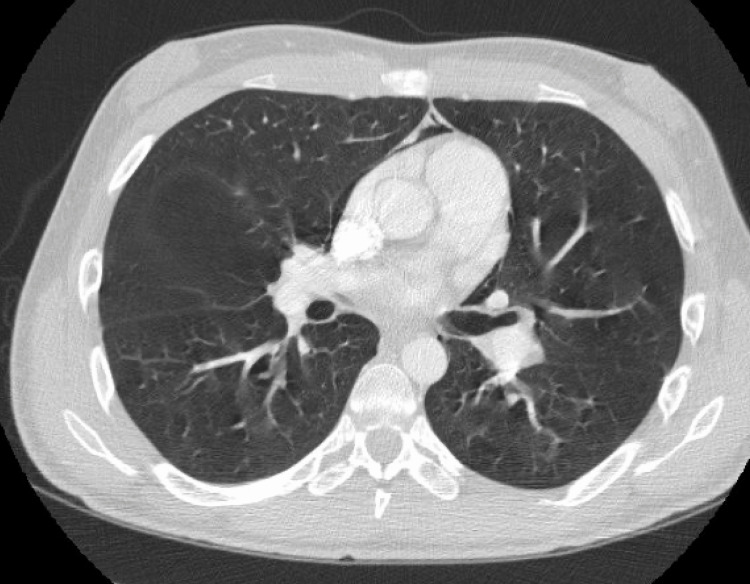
Computed tomography (CT) of the thorax pneumomediastinum with air present in the anterior and middle mediastinum.
